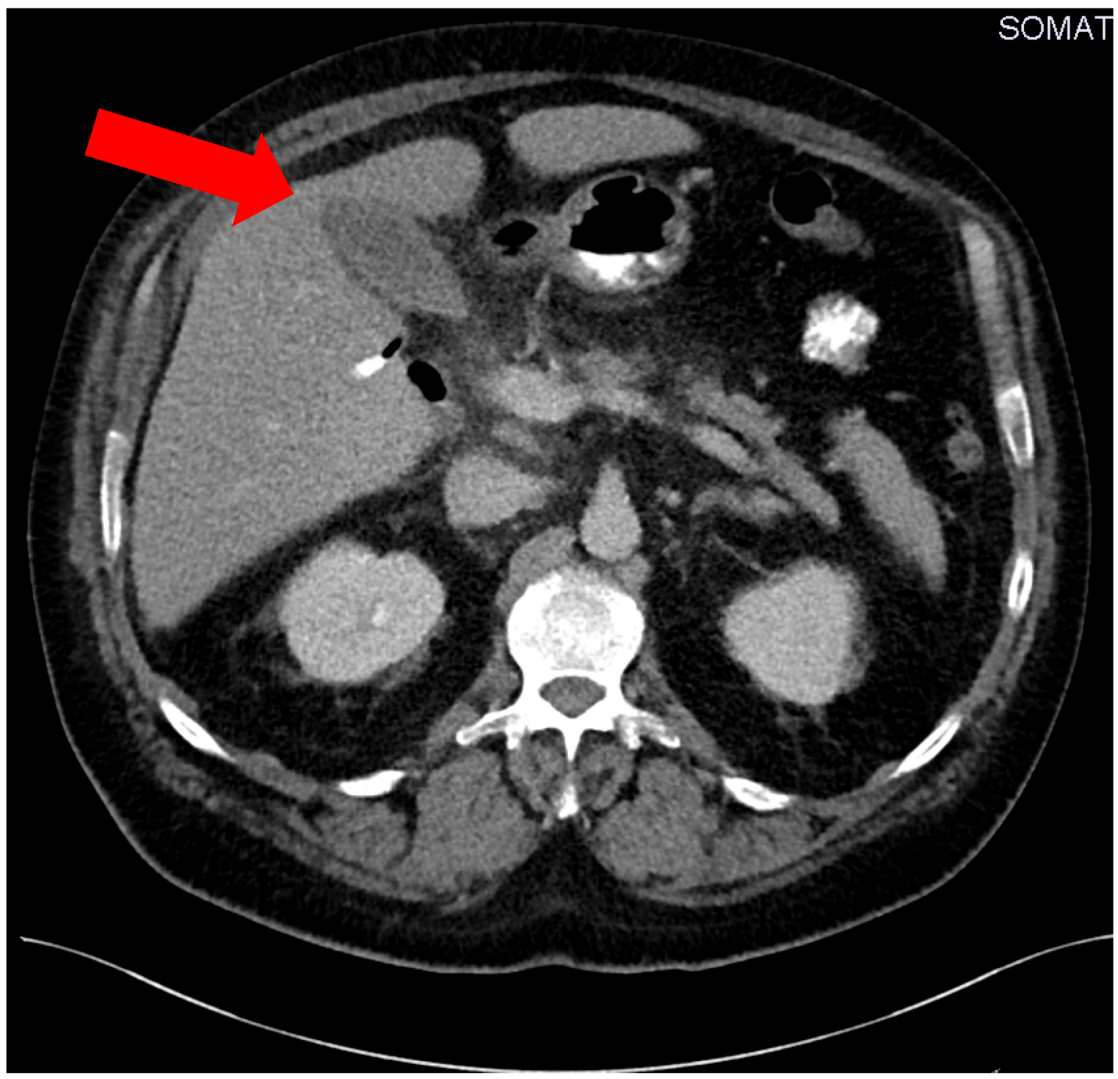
Preoperative computed tomography of the hepatic abscess (red arrow)
